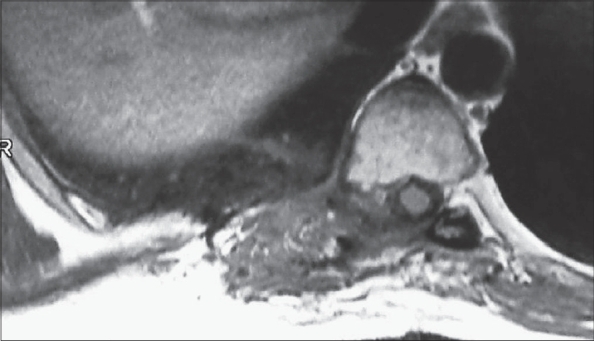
Postoperative T1 WI axial MRI showing resolution of cyst. The lamina and the right-sided pedicle and rib were surgically excised along with the cyst. There are no fluid-filled cystic areas. The size of the cord is restored
